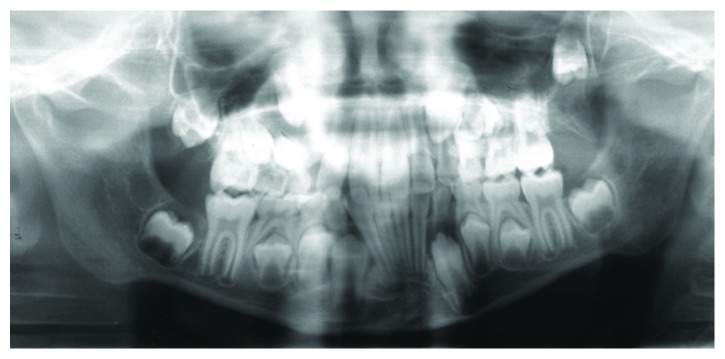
Panoramic radiograph (January 2012). Unclear radiolucency at teeth 13 and 23. Ectopic tooth 27.
